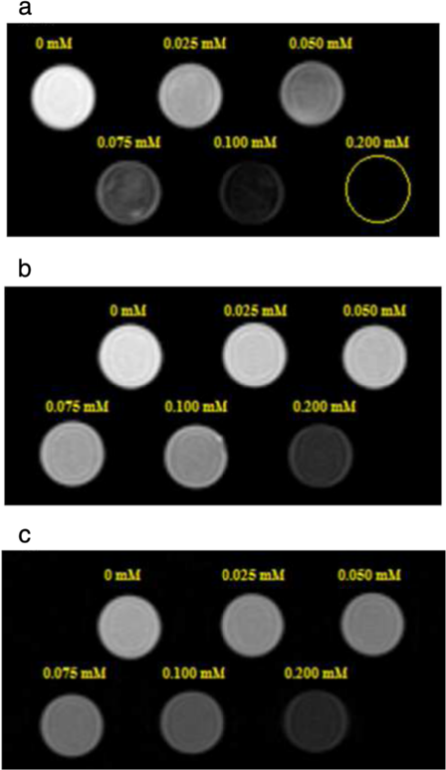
T_2_-weighted MRI images (1.5 T, spin-echo sequence: repitition time TR = 1600 ms, echo time TE = 108 ms) of the dextran coated SPIONs a, chitosan coated SPIONs b and mPEG-PCL coated SPIONs c at various iron concentration at 25 °C
